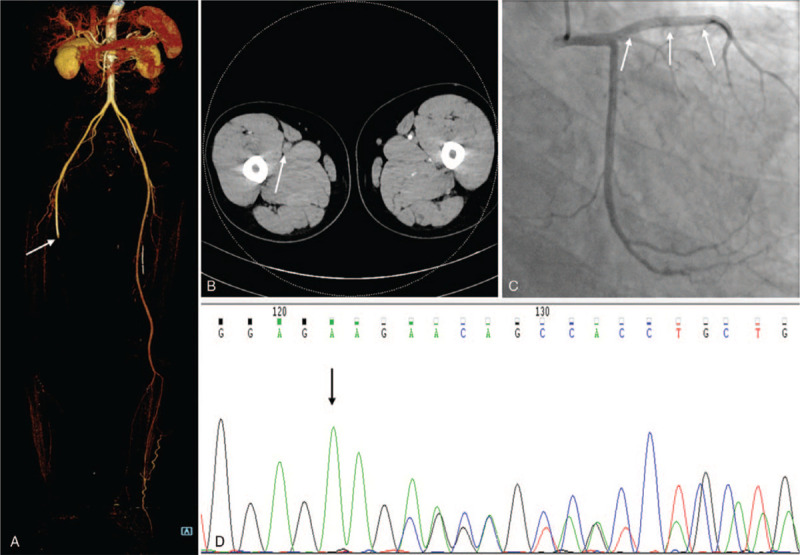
Preoperative examinations of the patient. (A and B) Lower extremity computed tomography angiography (CTA) demonstrated acute arterial embolism in the middle segment of the right superficial femoral artery (*white arrow*). (C) Coronary angiography showed a stent shadow (*white arrows*) in the proximal end of the anterior descending branch, and TIMI grade 3 in the distal blood flow. (D) Sequencing of the PROC gene exon 7 revealed that deletion mutation of 3 nucleotides AAG at 577 to 579 of the cDNA sequence led to the deletion of lysine 192 (*black arrow*).
